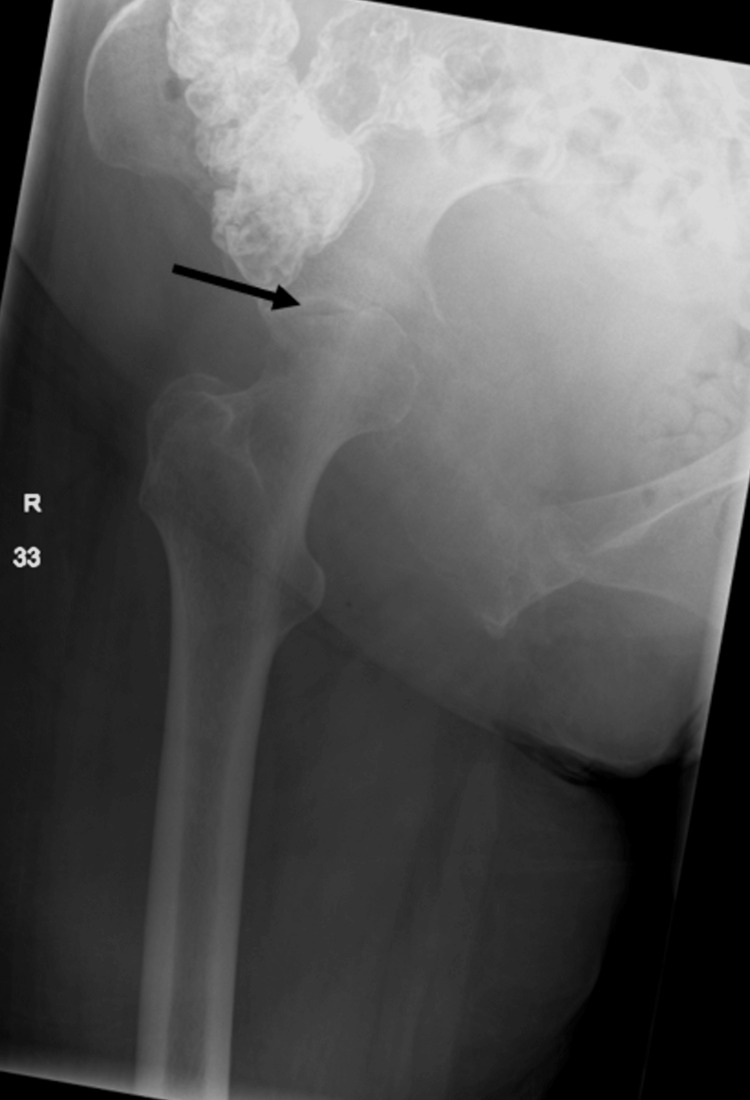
X-ray of right hip joint showing age-indeterminate deformity (arrow); may represent compression fracture vs. avascular necrosis
